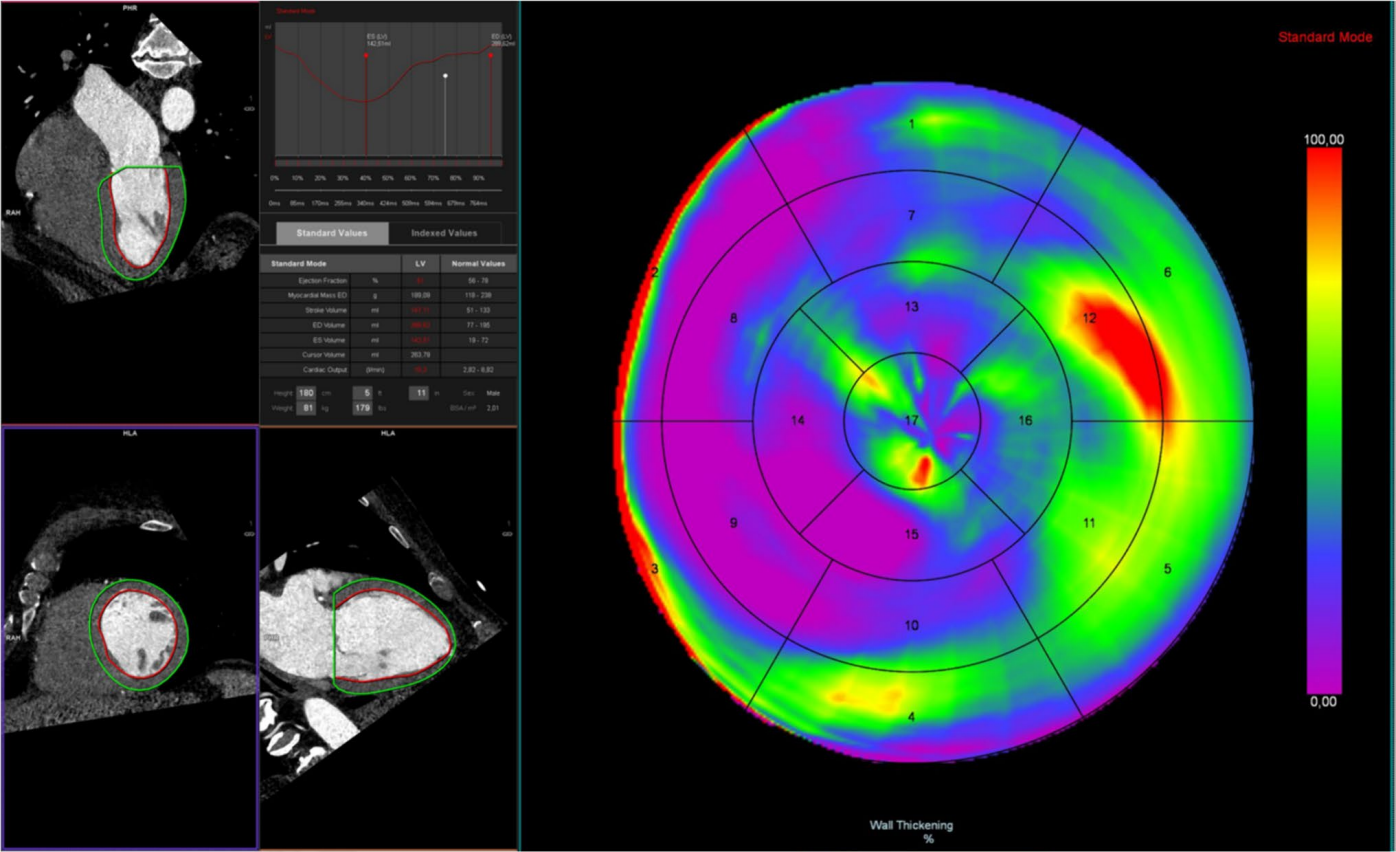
CT-derived volumetry and functional analysis. The images on the left describe the process of CT-based volumetry using endo- and epicardial contouring. Functional assessments allow the quantification of global cardiac function as well as in-depth visualization of wall-motion abnormalities as shown by the bullet plot on the right
